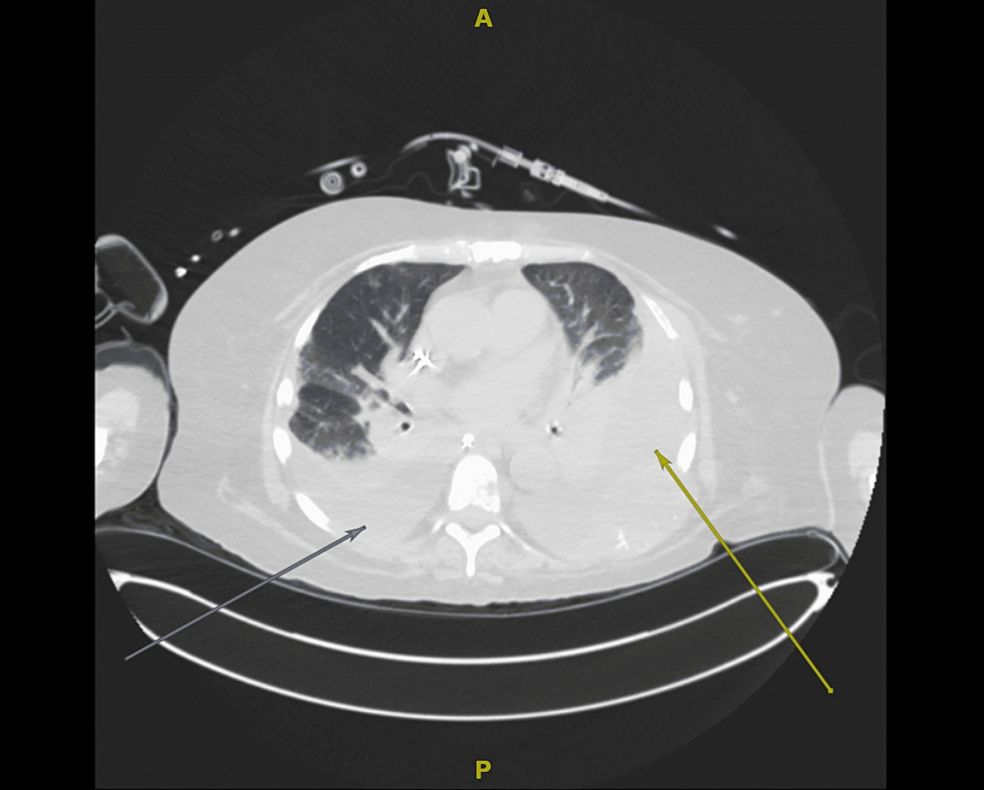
Axial section from a non-contrast CT chest showing bilateral pleural effusions (arrows) and ground-glass opacities
